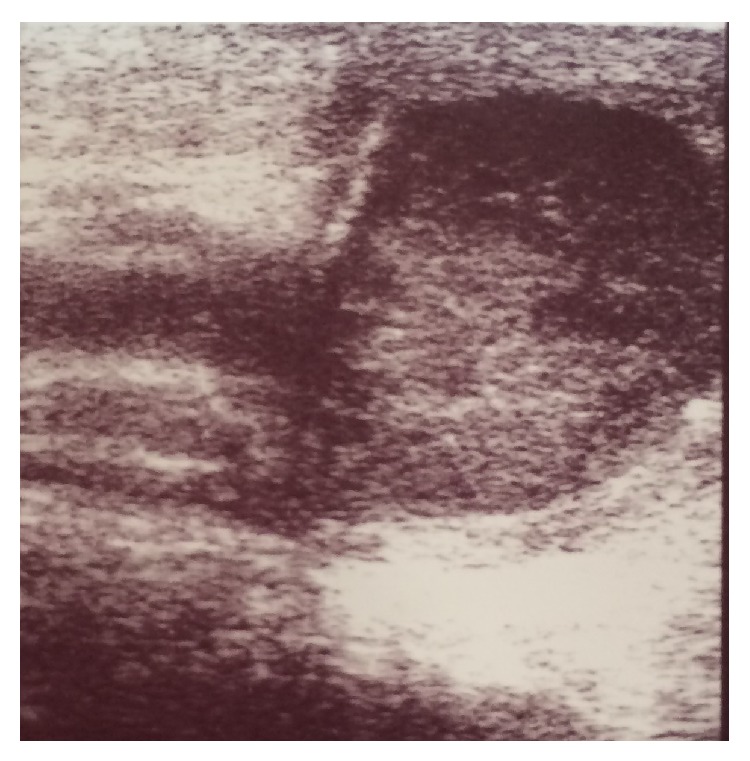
Ultrasound study shows the right testis with edematous appearance suggesting the presence of pyocele. This could be due to perforated appendicitis.
